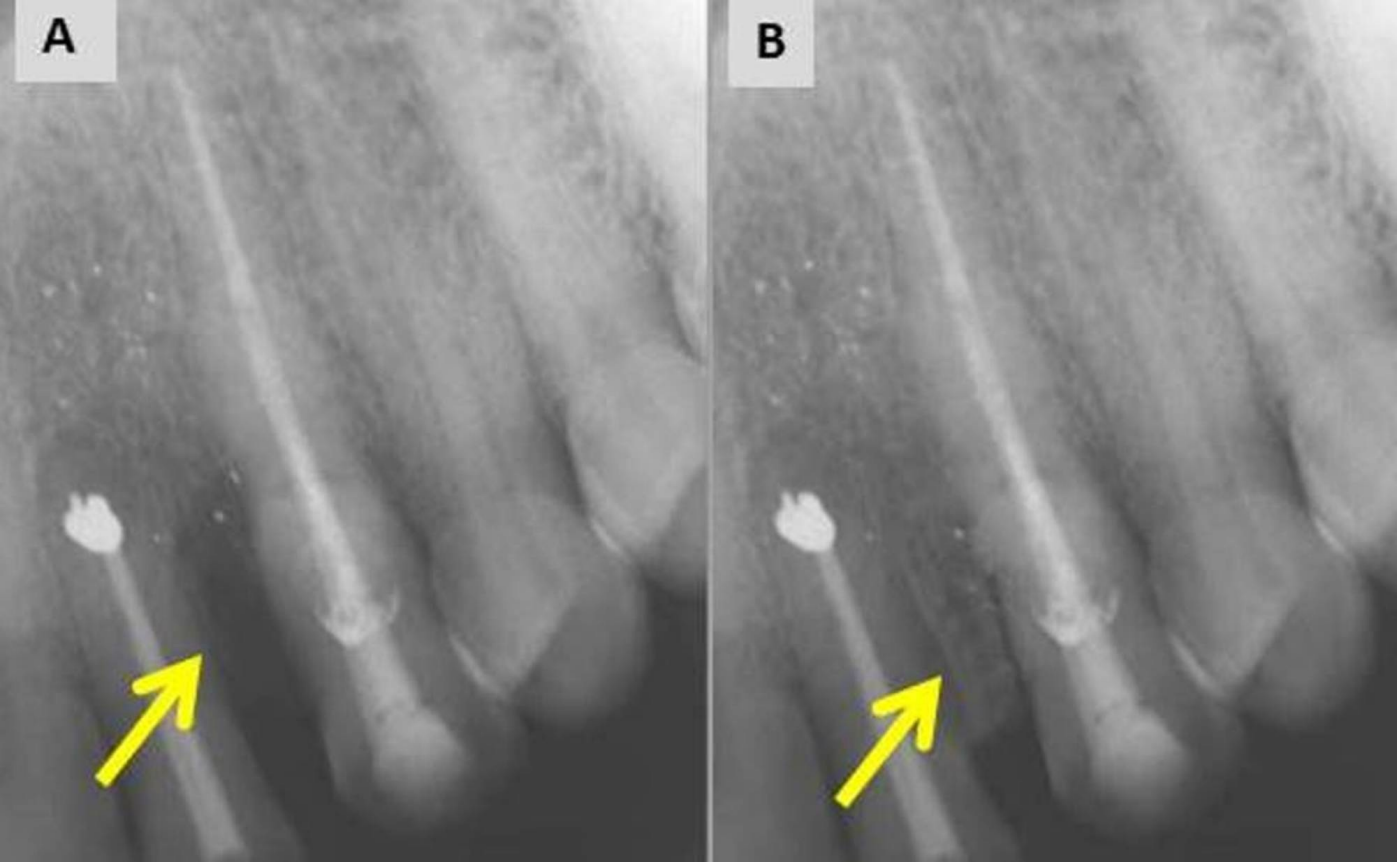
Postoperative and follow-up radiograph. (A) Post-operative radiograph. (B) Four-year follow-up radiograph showing satisfactory healing with bone formation.
